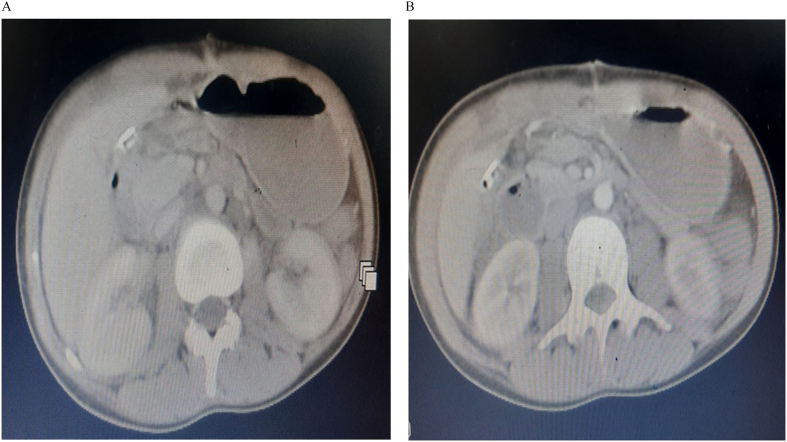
Post-operative abdominal CT scan of the patient.
